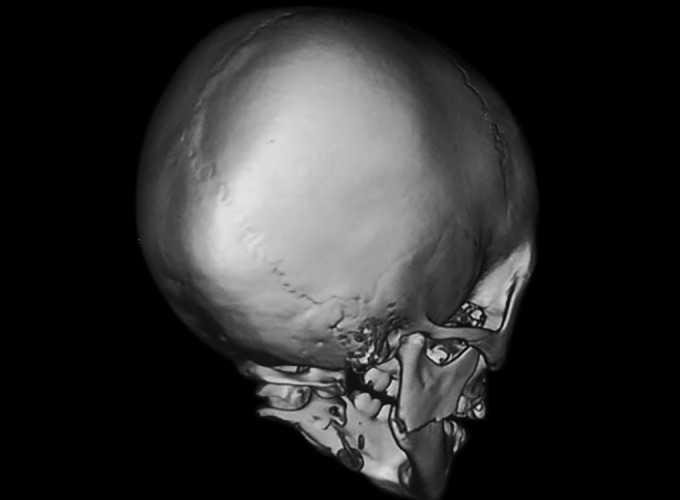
Three dimensional facial CT (arrowhead) depicting displaced right angle of mandible
